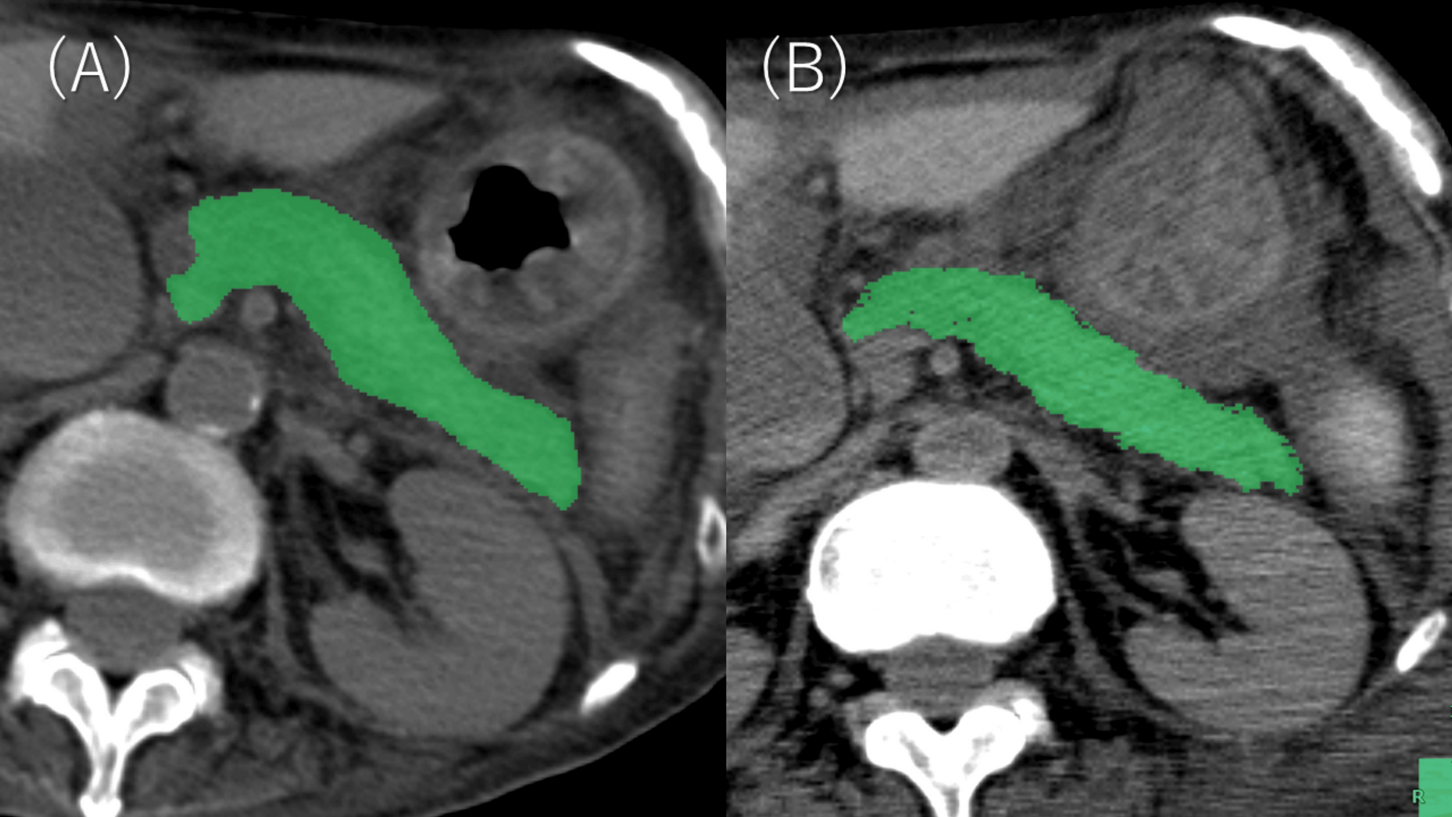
An example of regions of interest for the pancreatic parenchyma on antemortem and postmortem CT images. Regions of interest (highlighted in green) are overlaid on the whole pancreatic parenchyma in each CT slice, displayed on antemortem (A) and postmortem (B) CT images of the same patient.
